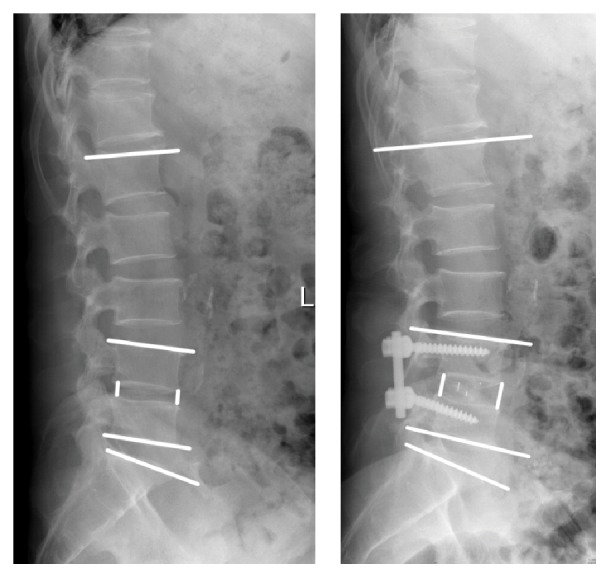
Preoperative and 3-day postoperative anterior-posterior X-ray images showing unilateral pedicle screw instrumented TLIF on L4-5 level. The angle of lumbar lordosis was measured between the superior endplate of L1 and S1. The functional spine unit was measured between the superior endplate and inferior endplate of fusion segment. The mean disc height was defined as the arithmetic mean between anterior and posterior disc height.
